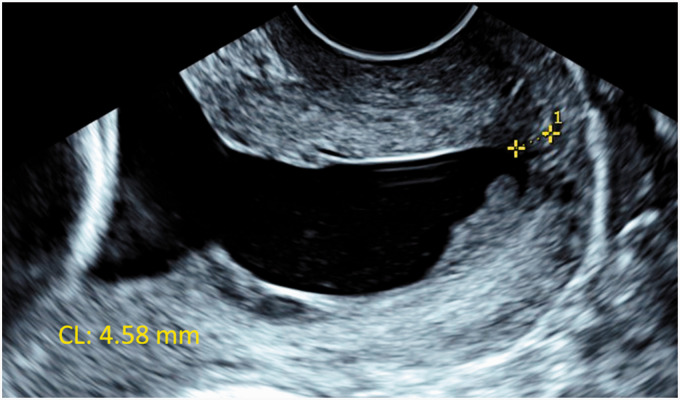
Transvaginal ultrasound of the cervix in longitudinal view at 22 weeks of gestation. The cervix was 4.58 mm long (yellow callipers), with a large funnel and intra-amniotic sludge.
